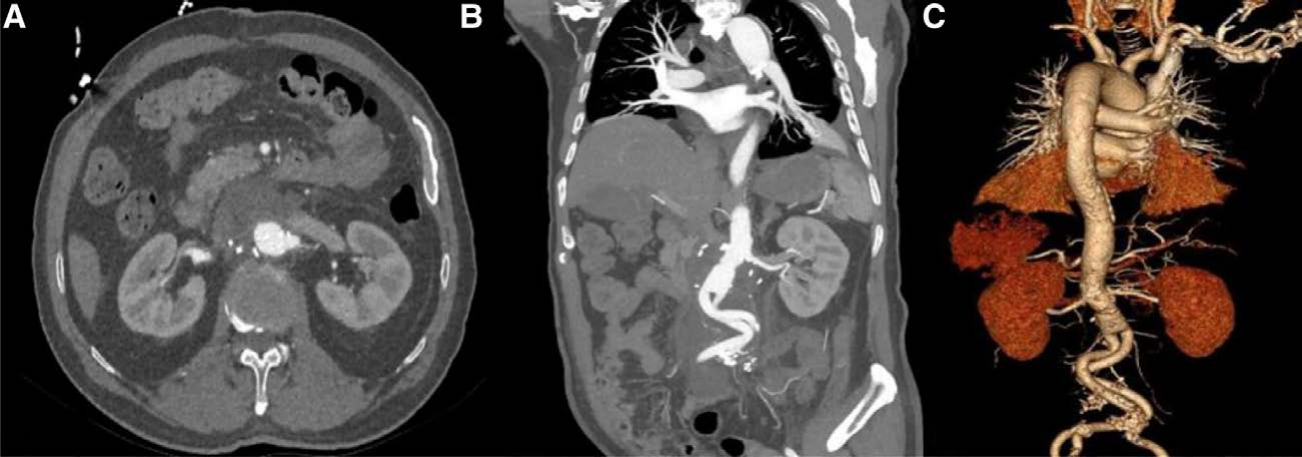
Postoperative computed tomography angiogram revealed an unobstructed vascular graft of abdominal aorta. A: Axial image of the abdominal cavity; B: Sagittal image; C: 3D reconstruction.
